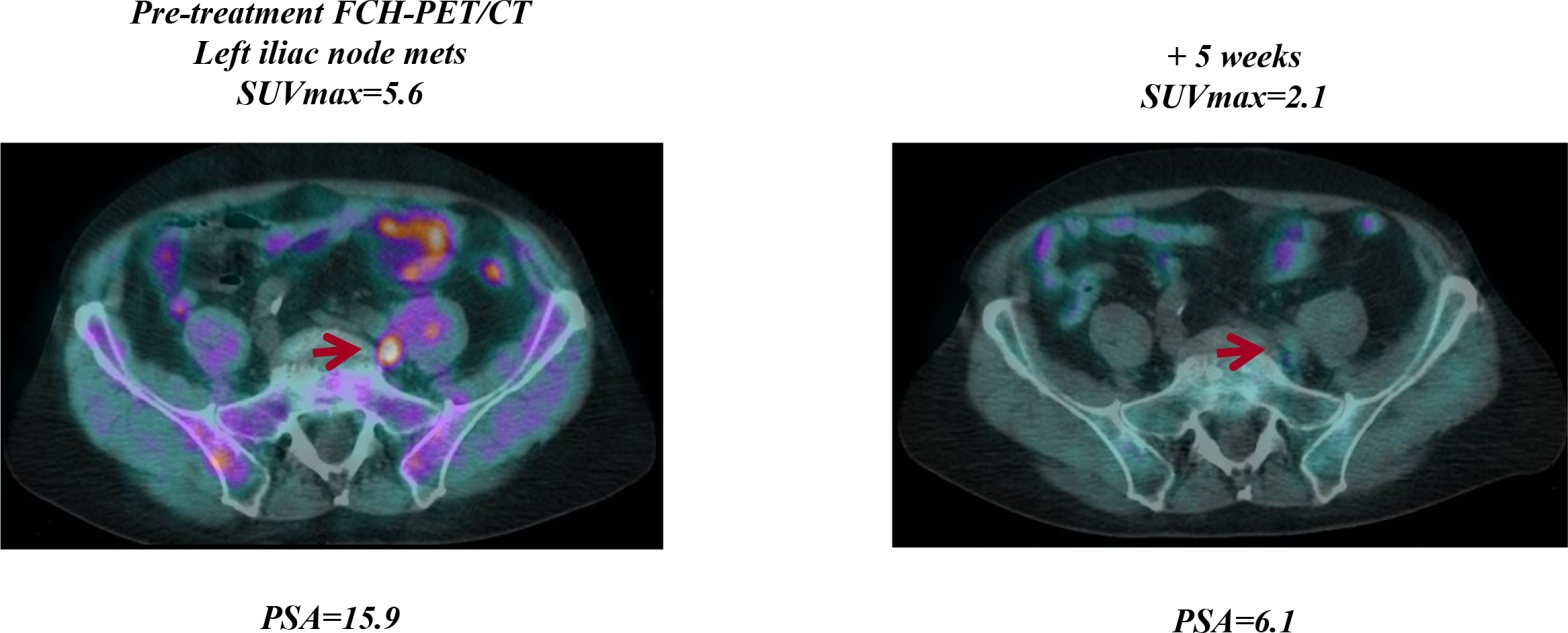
Early FCH-PET/CT complete response on abiraterone treatment Baseline FCH-PET/CT shows a single left iliac nodal lesion, 5 week FCH-PET/CT resolution correlated with PSA decline and then with undetectable PSA levels (PSA < 0.03 ng/mL) after 6 months of abiraterone therapy.
